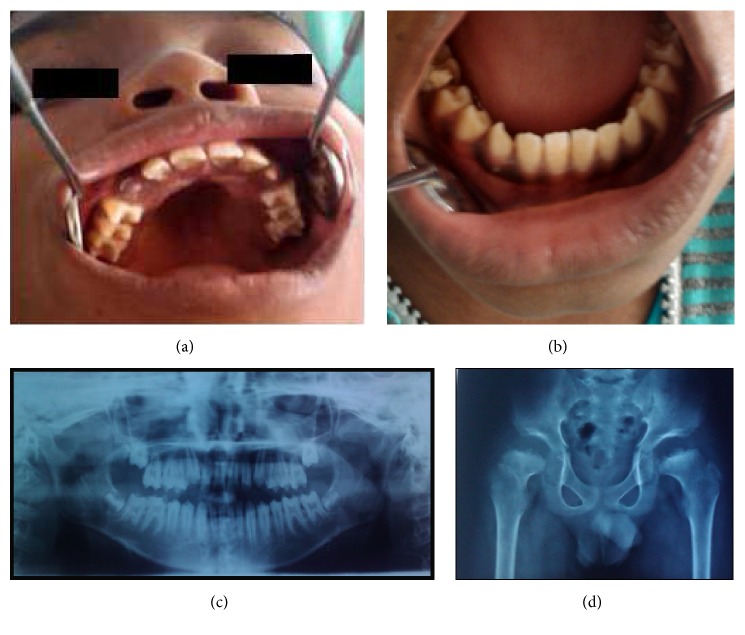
(a), (b) Spade-shaped incisors, pointed cusps, and spacing between teeth in both maxillary and mandibular teeth in case 2. (c) Orthopantomograph showing thin enamel with normal radio density. (d) Pelvic radiograph showing shallow acetabula and square-shaped femoral head in case 2.
